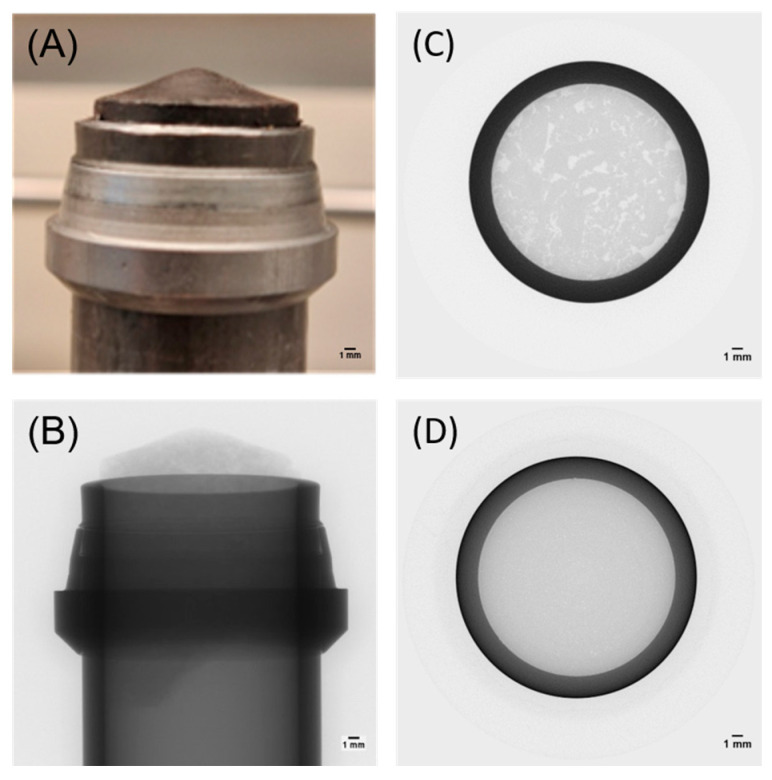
(A) The solid resin plug inside a steel tube, (B) the CT scan of (A), (C) a cross-section CT scan image of a sample with 10 wt.% initiator, and (D) a cross-section CT scan image of a sample with 0.5 wt.% initiator. The increased grayscale intensity corresponds to a higher density. The scale bar represents 1 mm.
